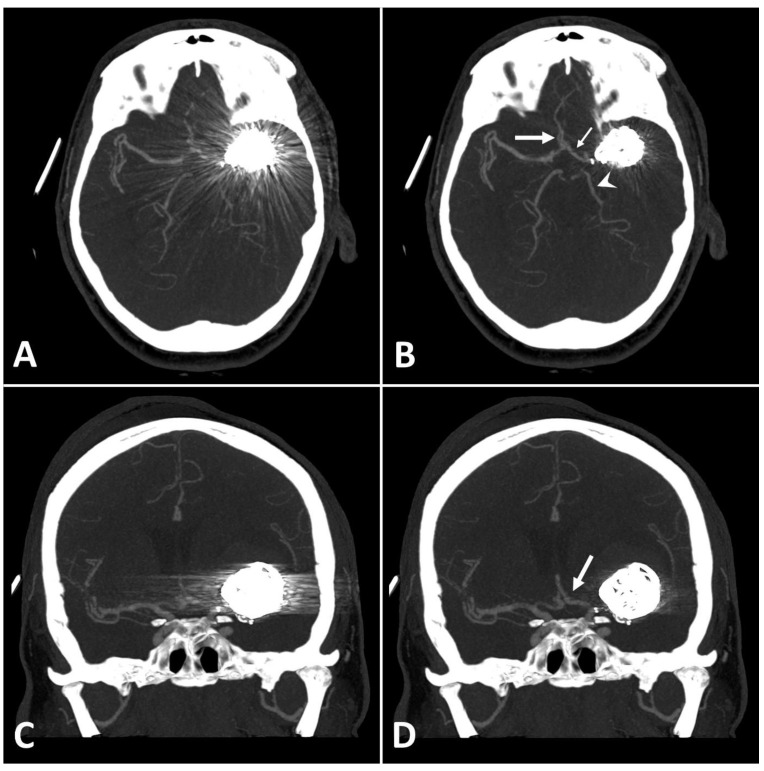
Exemplary follow up UHR-CT-angiography in axial and coronal plane without (A,C) and with SEMAR (B,D) of a 48-year-old female after intracranial coil-embolization of a left sided middle cerebral artery aneurysm, demonstrate the efficacy of SEMAR in visualizing vessels adjacent to the coil package like the ipsilateral middle cerebral artery (thick arrow in (B)), the anterior cerebral artery (thin arrow in (B)), the ipsilateral posterior cerebral artery (arrowhead in (B)), and the middle cerebral artery (thick arrow in (D)). All images were reconstructed as MIP (Maximum Intensity Projection) with 12.5 mm slice thickness.
